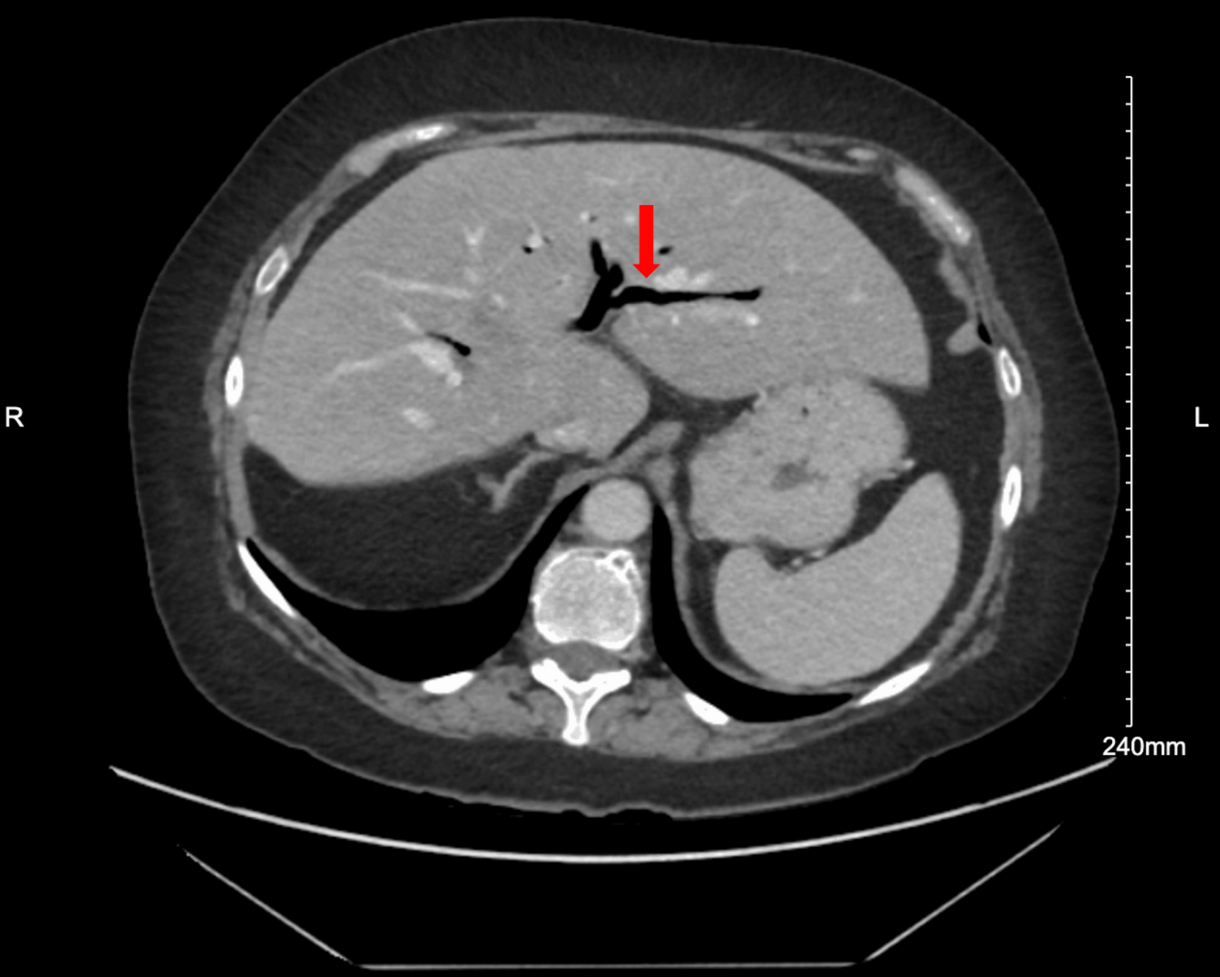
Axial computed tomography (CT) abdomen/pelvis with contrast. Arrow points to the pneumobilia.
